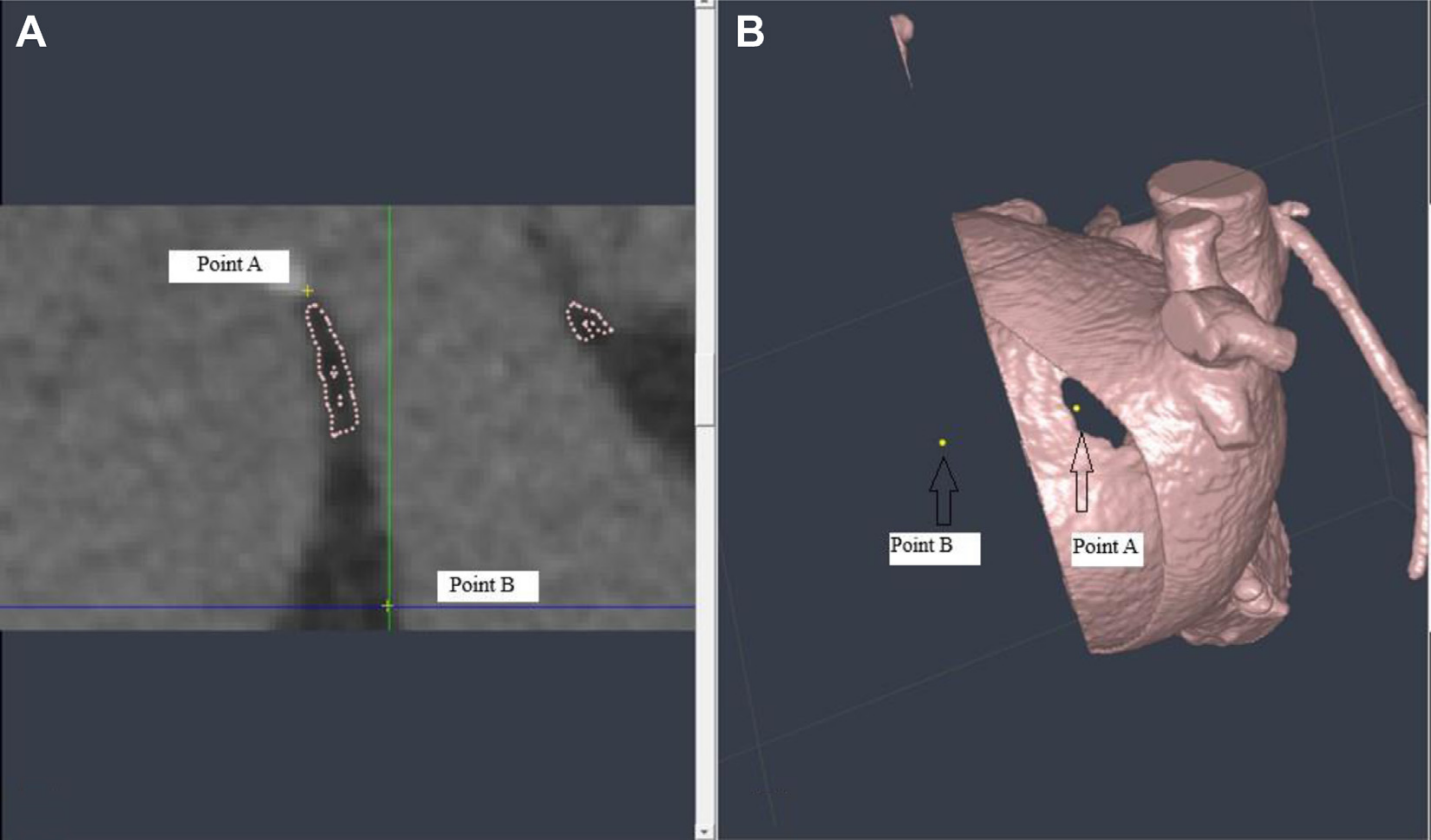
Combining CT Views and 3D Printing Model to Plan Surgery Combining the computed tomography scan image (A) with the 3-dimensional model image (B) to plan for surgery. It was decided to enlarge the opening to a circle with a diameter extending from point A to point B; therefore, we can open the obstruction and keep other structures away from injury. The distance between A and B is approximately 2.5 cm.
